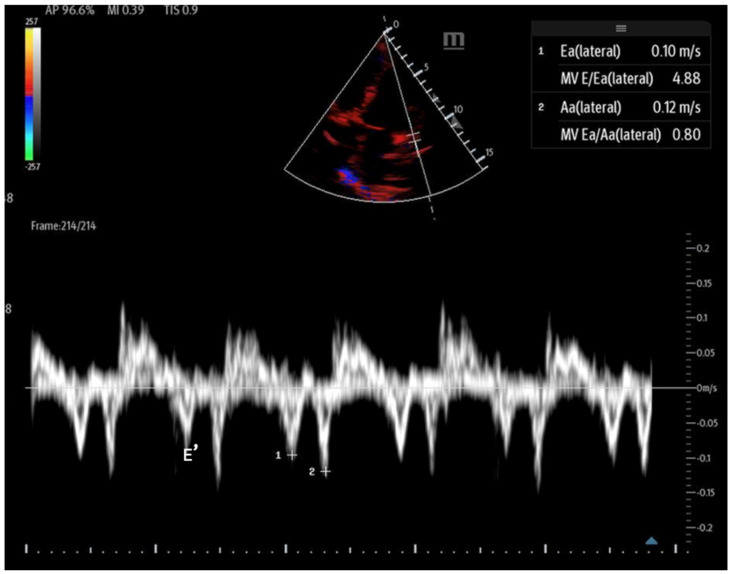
Tissue Doppler at the lateral septal annulus to record the E′ wave (labeled).
